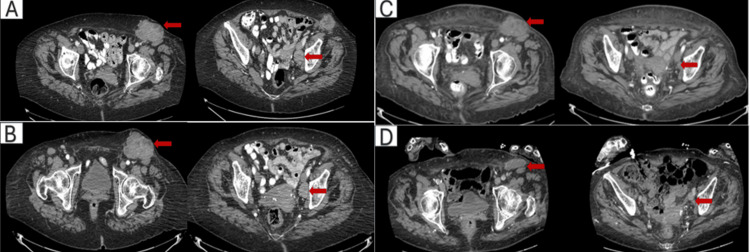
Difference in size of inguinal lymph nodes over course of treatment Axial CT images of the pelvis (A-D) demonstrating progressive reduction in left inguinal lymphadenopathy during treatment. Red arrows indicate representative nodes. (A) Baseline at diagnosis. (B) Eight months after diagnosis. (C) 21 months after diagnosis. (D) 40 months after diagnosis, showing residual nodes. Hormonal therapy was initiated at baseline, and radiation therapy was administered between months 10 and 11.
